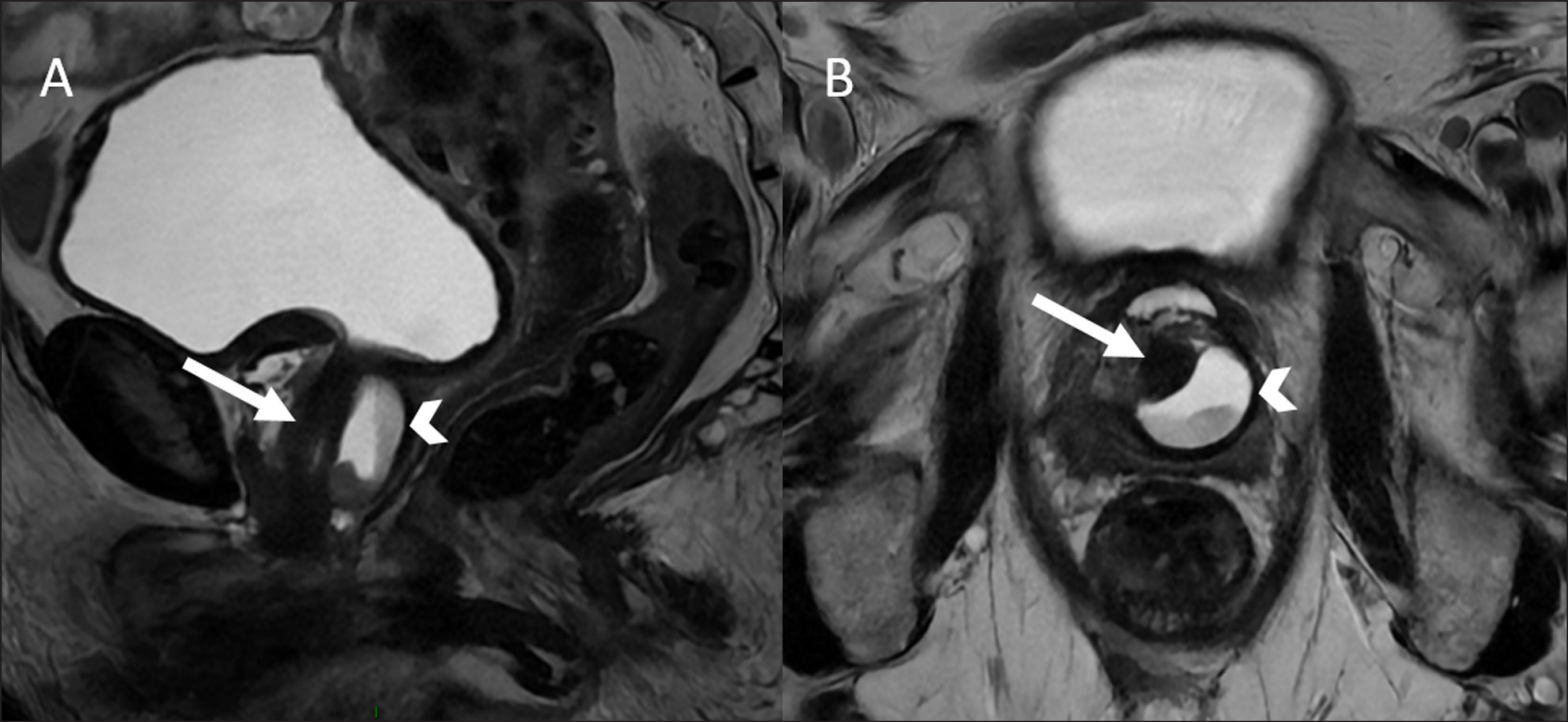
MRI also showed a solid part on the right posterolateral side of the diverticulum (Figure 2) characterized by a T2 slight hypersignal on T2-weighted images (Figure 2A, white arrow), enhancement on axial T1-weighted fat-suppressed images (Figure 2B, white arrow) with marked restriction diffusion (Figure 2C, white arrow) and low ADC value (1.051 × 10^–6^ s/mm^2^) (Figure 2D, white arrow). These characteristics are highly suspicious of intra-diverticular malignant transformation.
